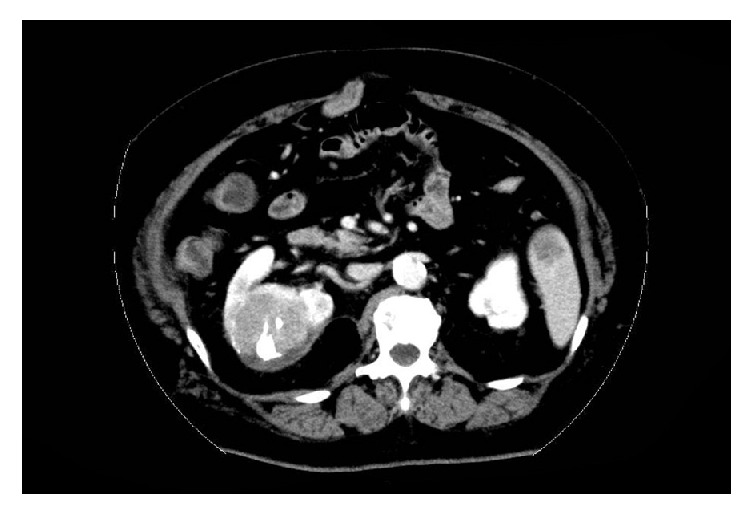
Follow-up CT, performed in September 2015. Marked early enhancement on arterial phase and wash-out on late portal phase indicate growing residual viable lesion.
